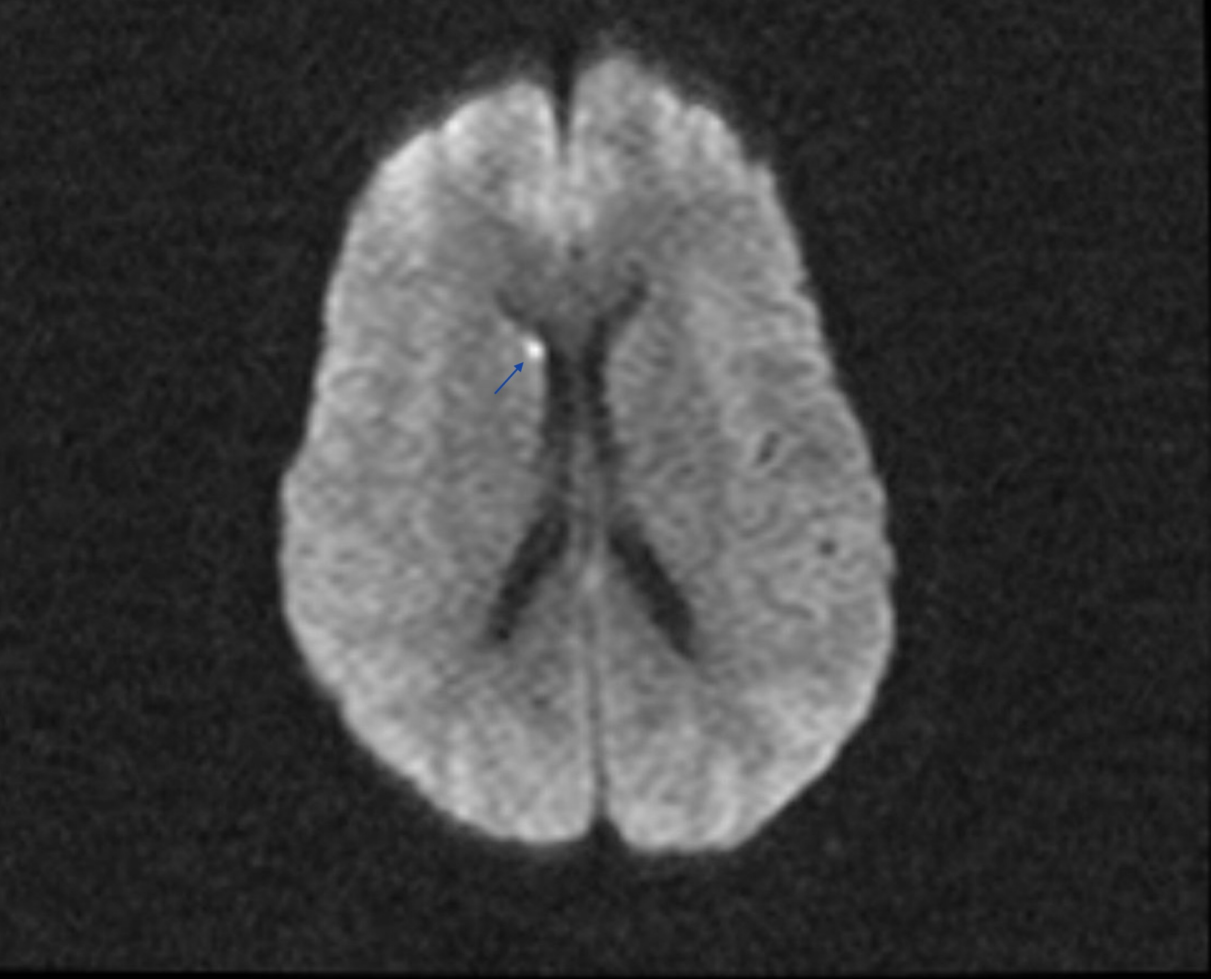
MRI brain with contrast Blue arrow points to the area of acute infarct.
